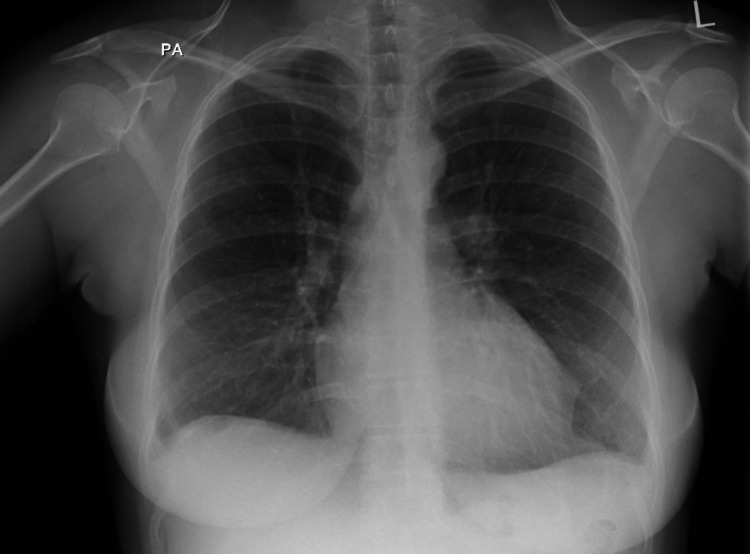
Chest X-ray three months after discharge. Chest X-ray taken three months after discharge from hospital demonstrating complete resolution of changes demonstrated earlier.
